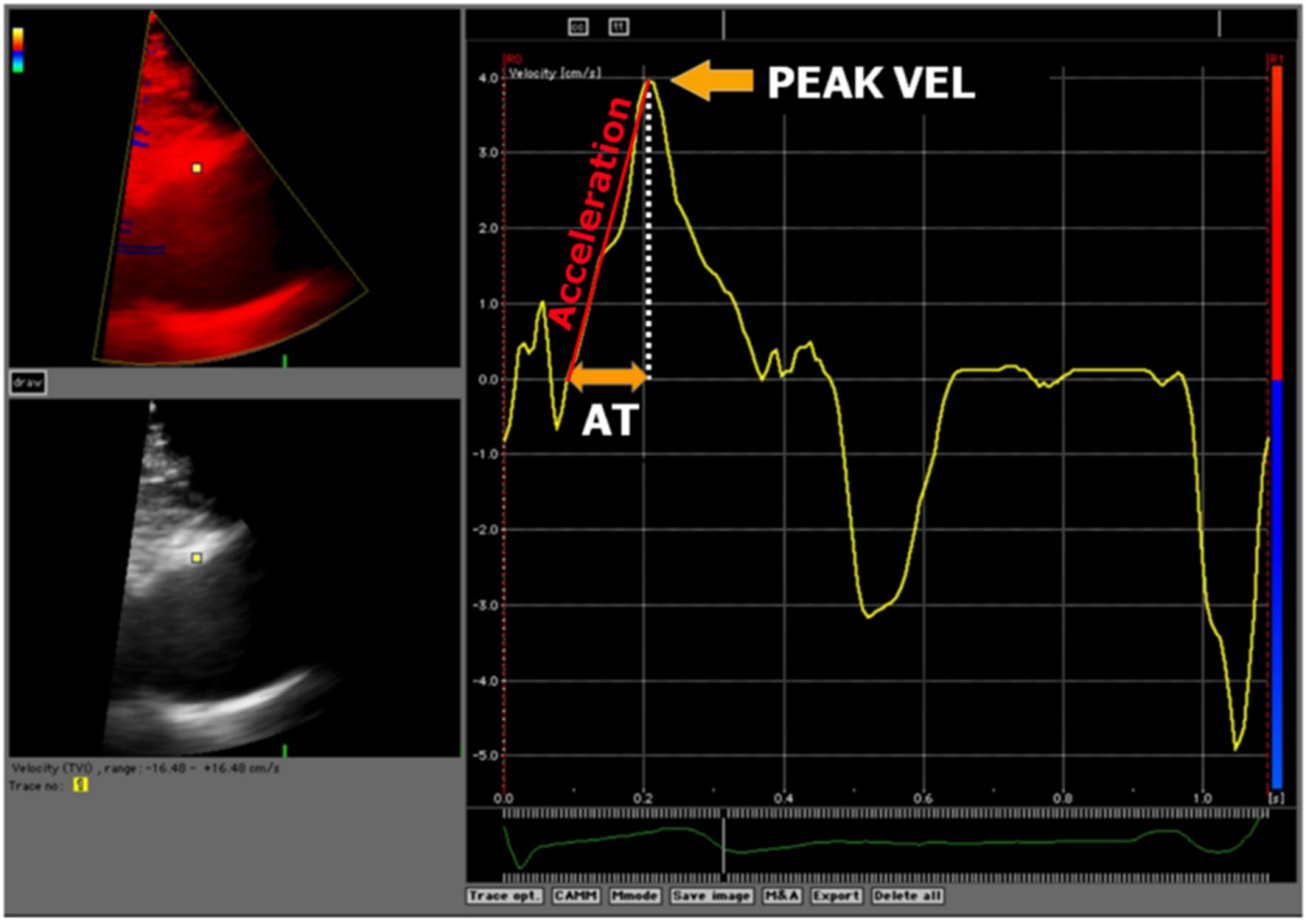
Representative radial velocity curve of the anterior ascending aortic wall recorded by tissue Doppler imaging. Velocity curve was obtained from two-dimensional, color tissue Doppler image of the ascending aorta in the parasternal long-axis view. The single arrow indicates peak systolic velocity of the anterior aortic wall. Oblique red Line represents acceleration. *AT* acceleration time
